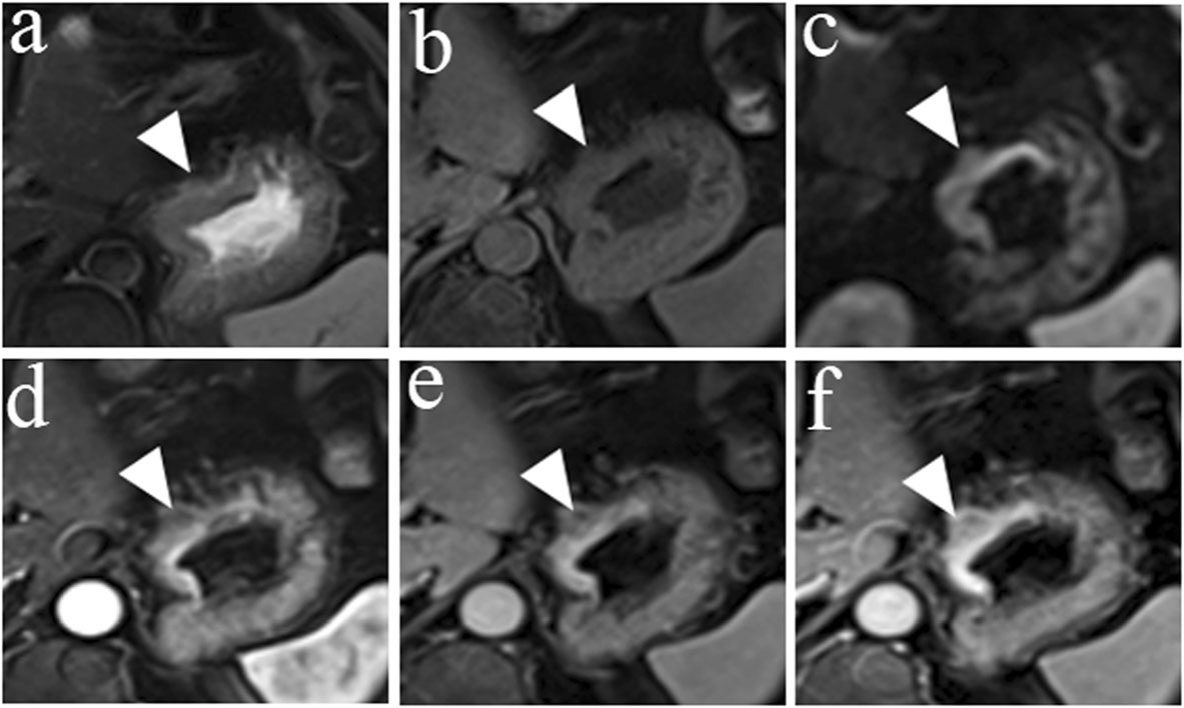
A 68-year-old man with esophagogastric junction cancer was pathologically diagnosed using conventional MRI as having stage T3, Sierwert II. a The axial T2W image shows the thickening and slight hyperintensity of the cardia wall and the peripheral adipose tissue was clear. b The axial diffusion-weighted image with b = 800 s/mm^2^ shows hyperintensity in the cardia wall. c A thickening pattern in the cardia wall is shown on an axial T1W image. d–f Compared with the adjacent normal tissues, the lesion was markedly enhanced on the arterial, venous and delayed phases of enhanced T1W images
